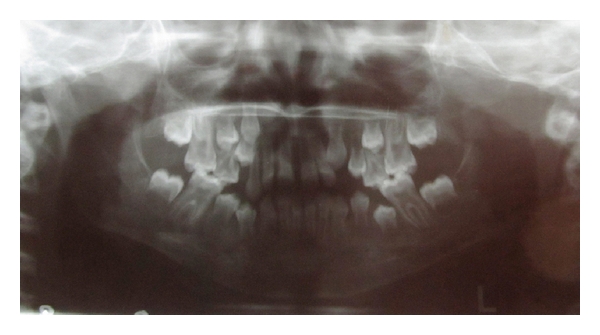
Showing orthopantomogram with apical involvement of 36 and 46.
